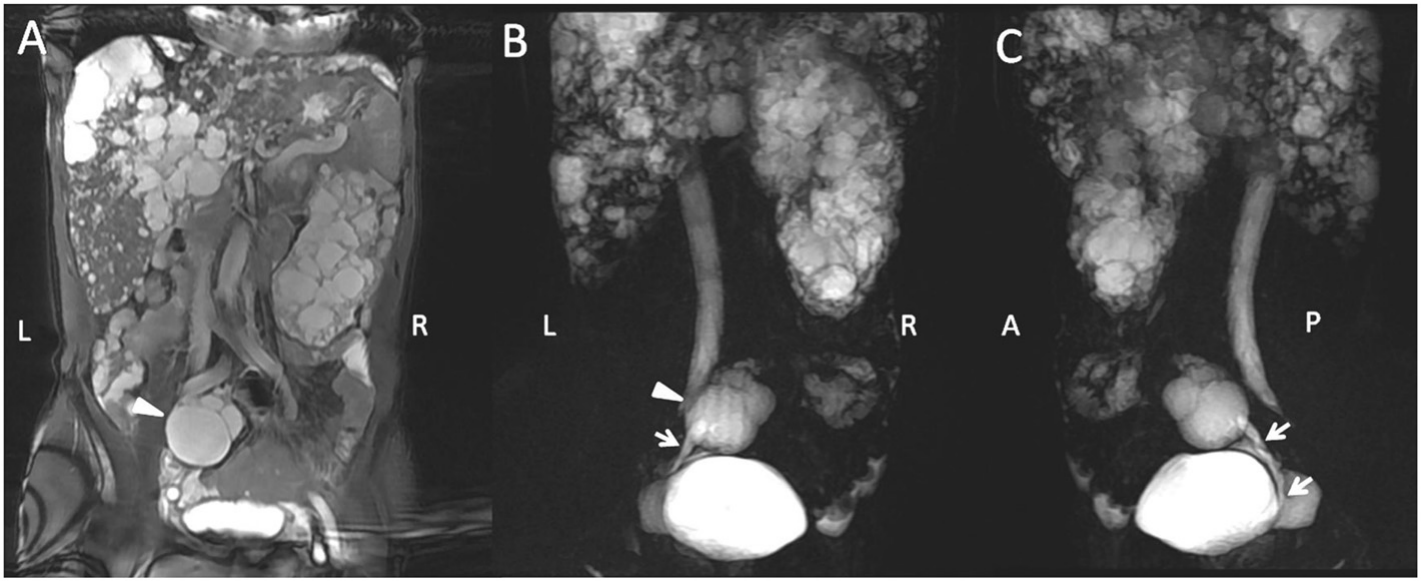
MRI scans showing a solitary left polycystic kidney with contralateral MCDK in Case 1 patient. (Triangle): right ectopic MCDK; (arrow): right ureter.
